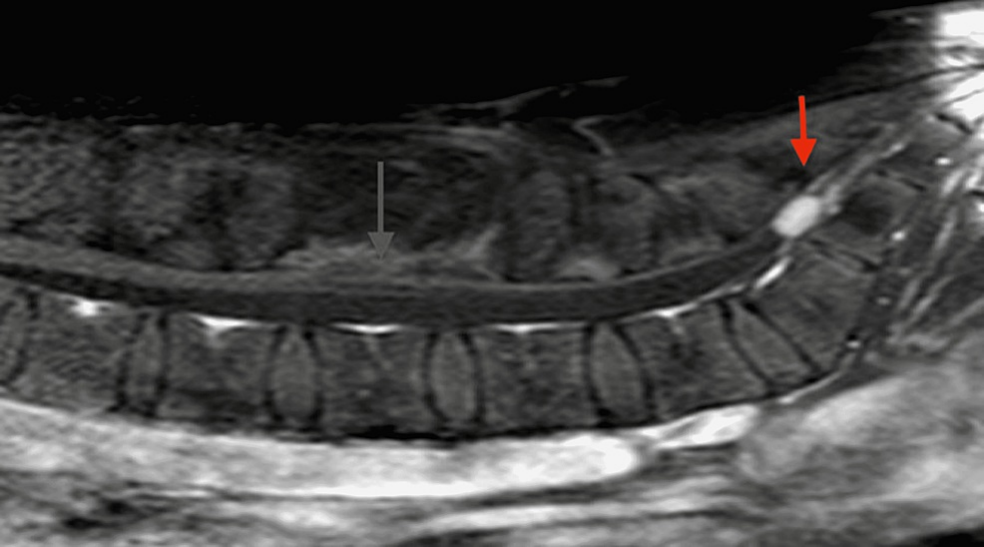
Sagittal post-operative T1 Gd+ MRI Sagittal post-operative T1 Gd+ MRI showing complete removal of the tumour at L3 (grey arrow) and increase in the size of the smaller one at S2 (red arrow), at the time of patient’s coccygodynia relapse.
